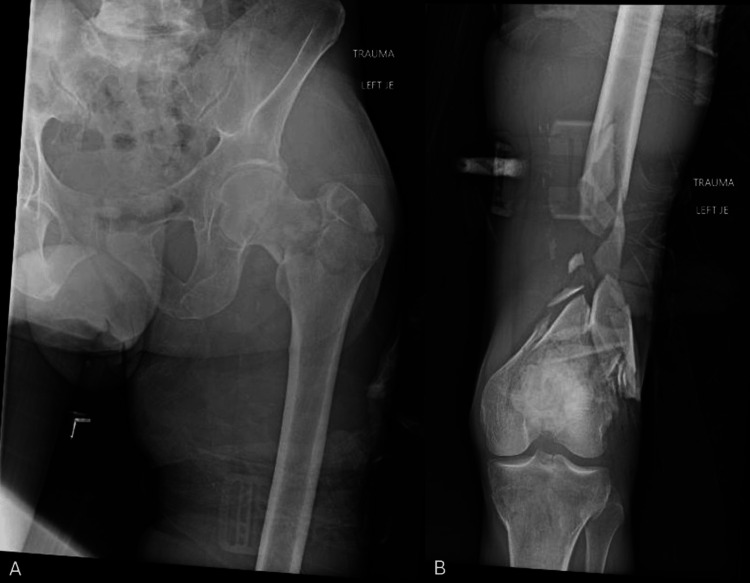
Distal femur fracture with segmental bone loss AP proximal femur (A) and AP distal femur (B) radiographs demonstrating a comminuted distal femur fracture with associated bone loss and an ipsilateral intertrochanteric femur fracture.
